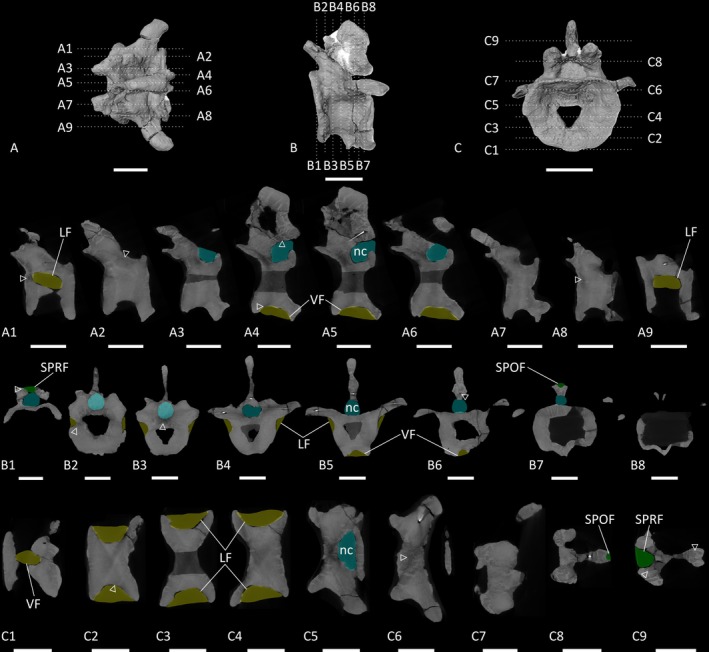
CT scan of *Giraffatitan brancai* caudal vertebra 7 MB.R.5000.6. (A–C) 3D rendering (A) dorsal view, (B) left lateral view, (C) anterior view; (A1–A9) CT scan slices in parasagittal cross‐section, (B1–B8) CT scan slices in transverse cross‐section, (C1–C9) CT scan slices in horizontal cross‐section. LF, lateral fossa; nc, neural canal; SPOF, spinopostzygapophyseal fossa; SPRF, spinoprezygapophyseal fossa; VF, ventral fossa. Key: Blue highlight, neural canal; green highlight, neural spine fossa; yellow highlight, centrum fossa; white arrow, pneumatic bone texture. Scale bars = 100 mm.
